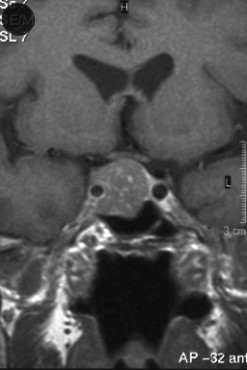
MRI of Pituitary – on admission.
